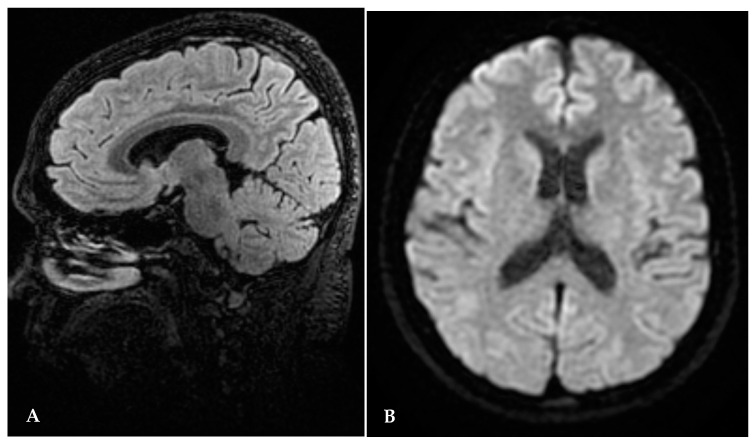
(A,B) Brain MRI seven months after thiamine start: disappearance of abnormal signal.
